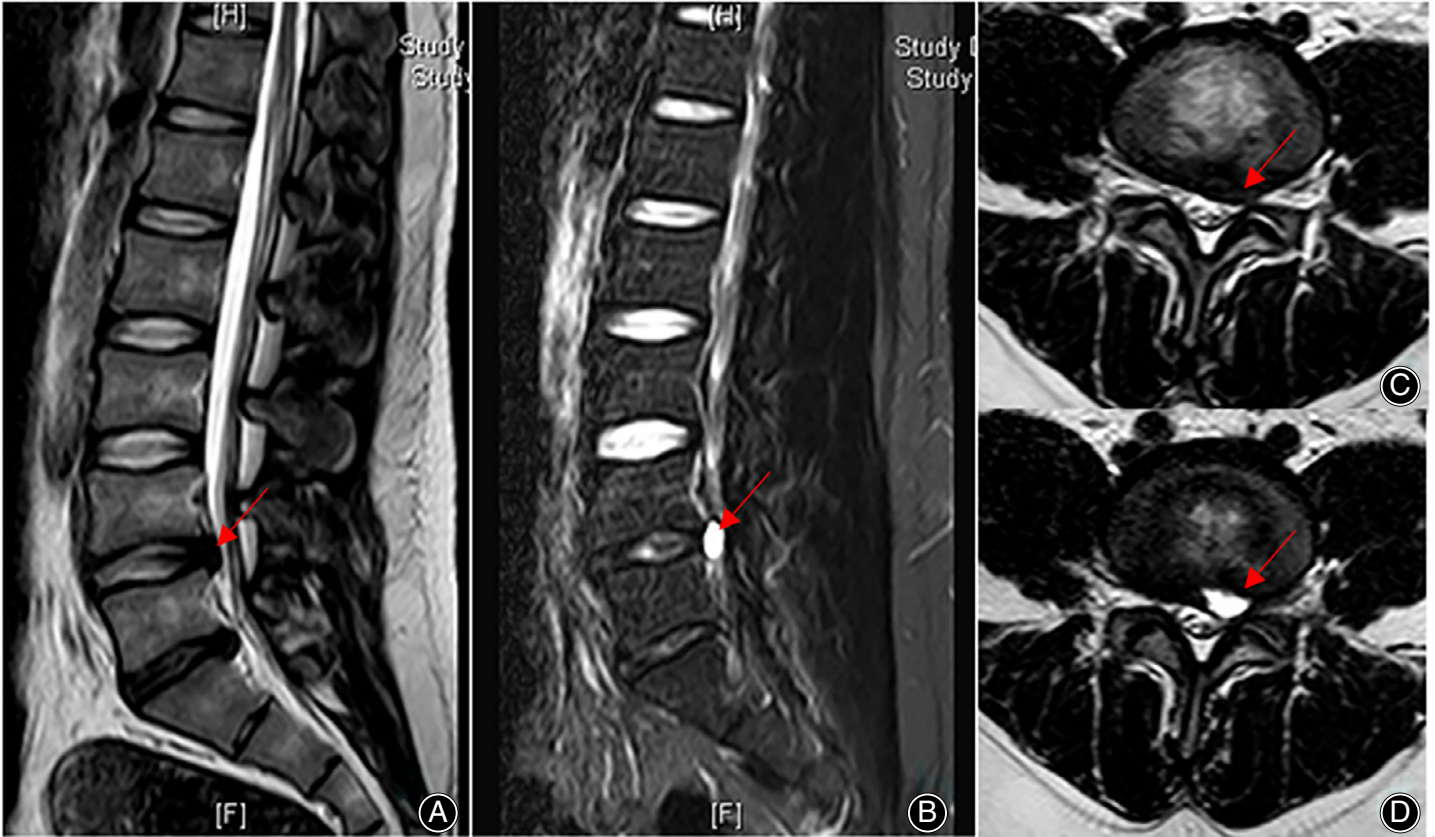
Lumbar MRI: disc degeneration (Pfirrmann grade II) and PDP. A\C: disc degeneration before lumbar discectomy, B\D: PDP (L5/S1 left).
